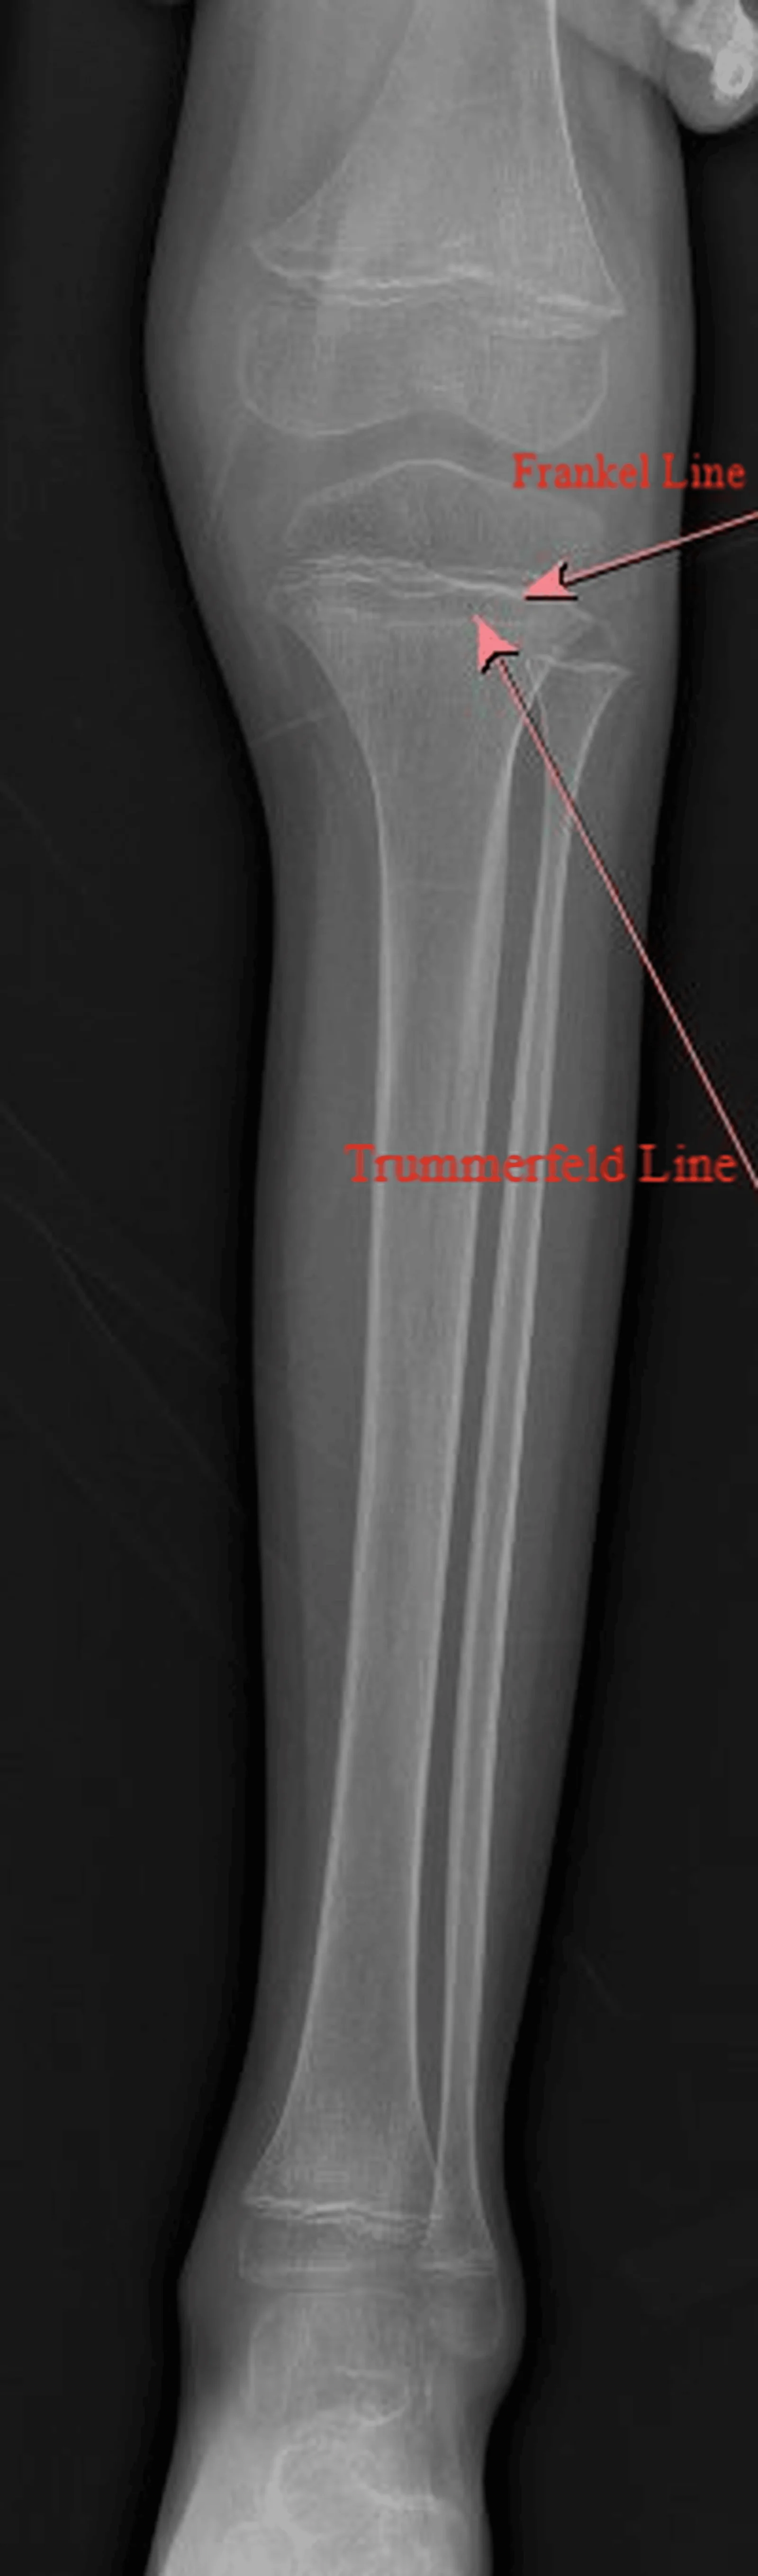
Plain radiographs of the lower extremities demonstrating diffuse osteopenia with a dense provisional zone of calcification (Frankel line, top arrow) and the adjacent lucent Trümmerfeld zone (bottom arrows), classic radiographic findings of pediatric scurvy.
